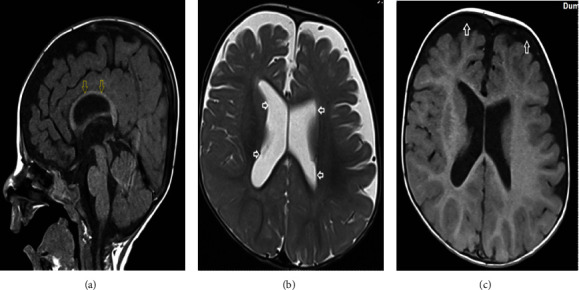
Brain MRI of a 10 months old boy in sagittal T1-WI (a) showing diffusely thin corpus callosum (yellow arrows), axial T2-WI (b) showing mildly dilated lateral ventricles (white horizontal arrows), and axial T1-WI (c) showing dilated subarachnoid spaces (white vertical arrows).
